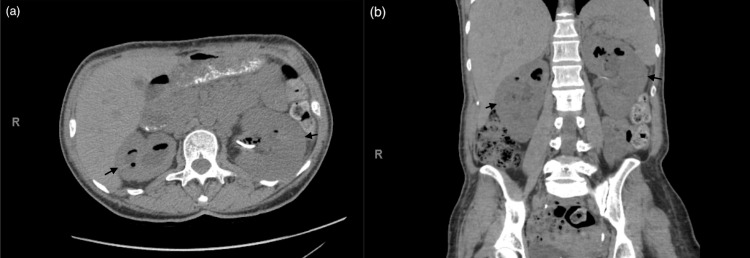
Non-contrast axial (a) and coronal (b) CT of abdomen and pelvis showing recurrence of bilateral emphysematous pyelonephritis.
